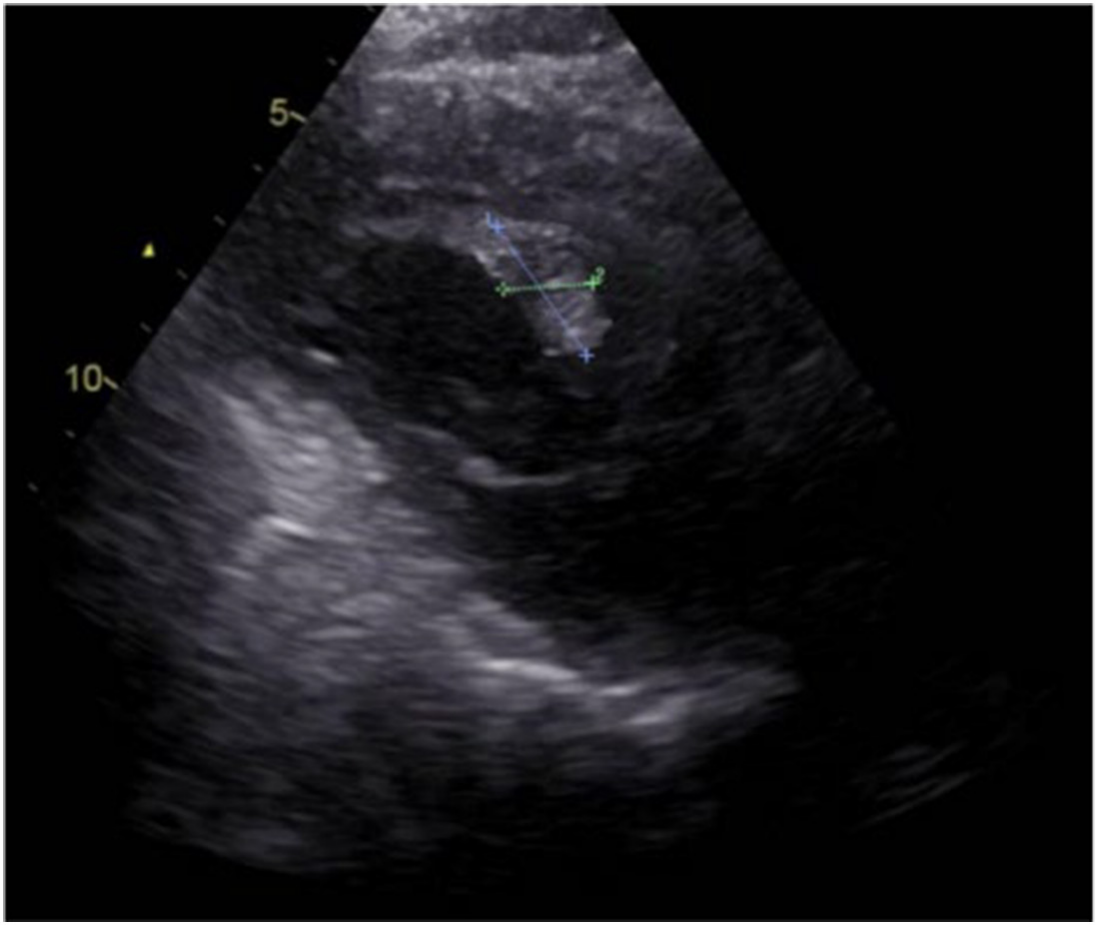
TTE of LV; 6 months after treatment with albendazole and praziquantel in cardiac hydatid cyst. The cystic area decreased in size (2.3 × 2.1 cm), with some evidence of increased echogenicity suggestive of calcification or fibrosis. There are no apparent regional wall motion abnormalities, indicating preserved LV function. LV, left ventricle; TTE, transthoracic echocardiography.
